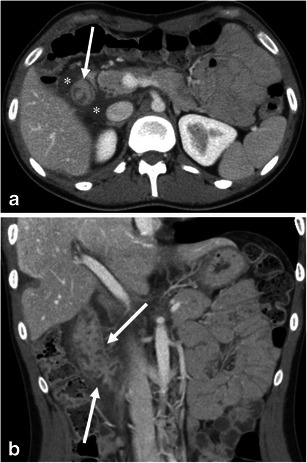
A 26-year-old female with HAE involving the 2nd portion of the duodenum. a The axial CE-CT shows the duodenal wall to be thickened (*arrow*) with surrounding free fluid (*asterisks*). b The coronal reformatted image shows oedematous thickening of the duodenal folds (*arrows*)
